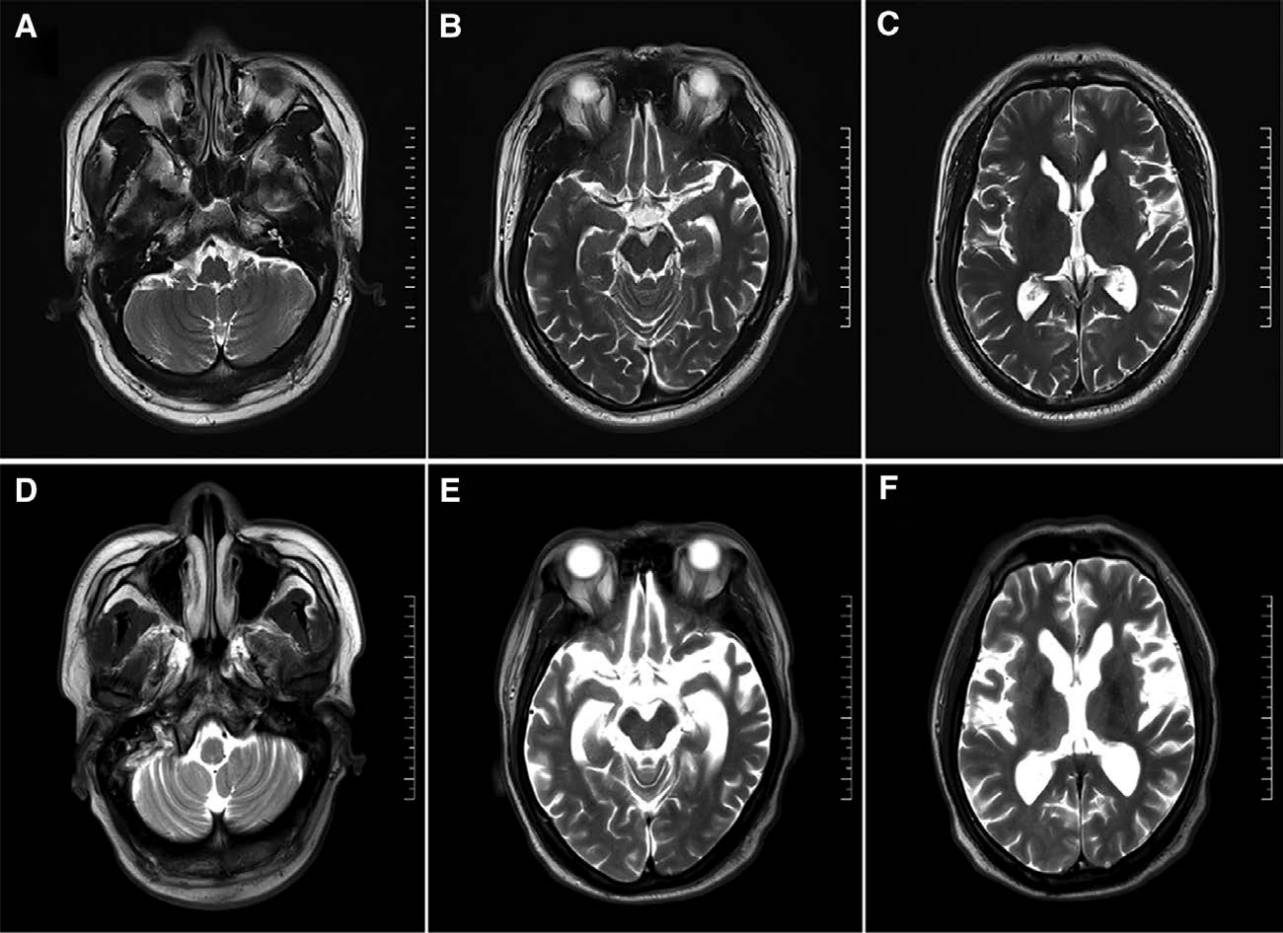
Brain magnetic resonance imaging (MRI) examination of patient. (A–C) T2 sequences demonstrated mild cortical atrophy at 2 years of onset. (D–F) T2 sequences demonstrated general brain atrophy, especially in lobes cortex and hippocampus at 6 years of onset. At 2 years post-onset, the Global Cortical Atrophy score was 1 bilaterally in the frontal lobes, increasing to 2 at 6 years; at 2 years, the score was 1 bilaterally in the temporal lobes, increasing to 3 at 6 years.
